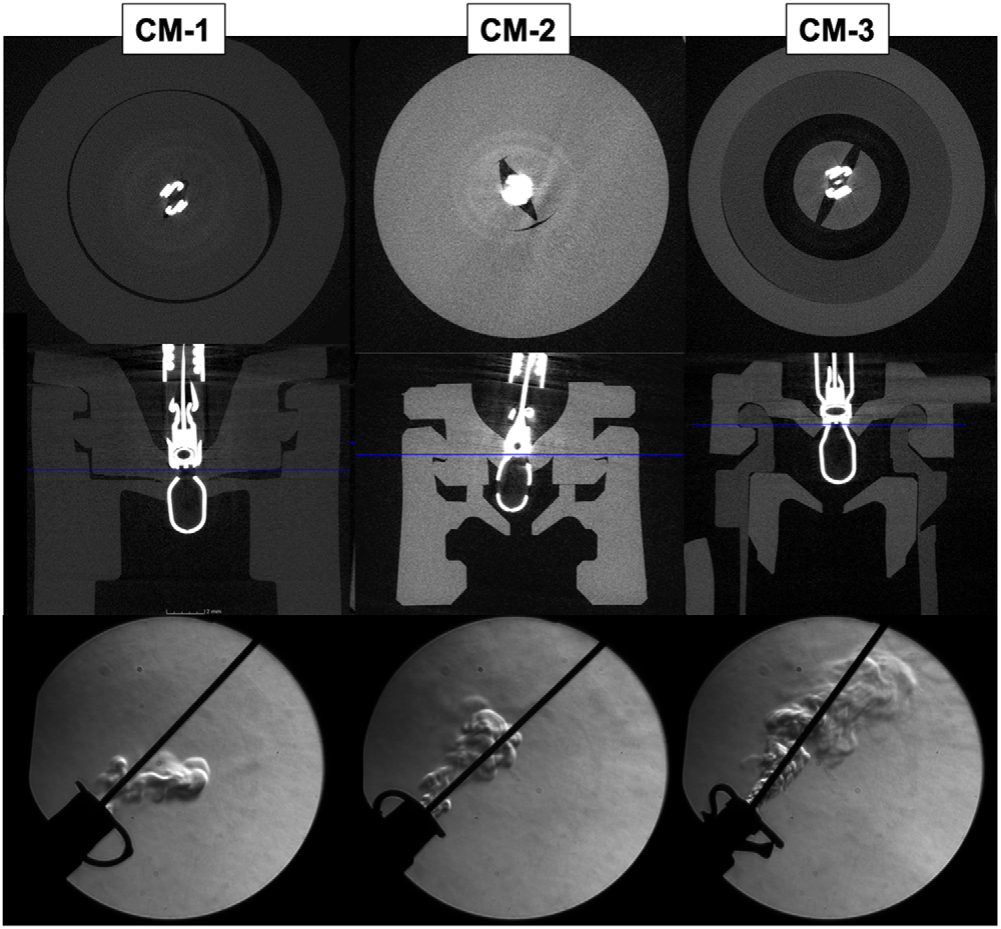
Non‐destructive examination for biopsy plugs using microfocus computed tomography and: Upper: axial view; Middle: coronal view; Lower: a typical image of gas leakage captured by the Schlieren system when forceps are inserted into each plug.
